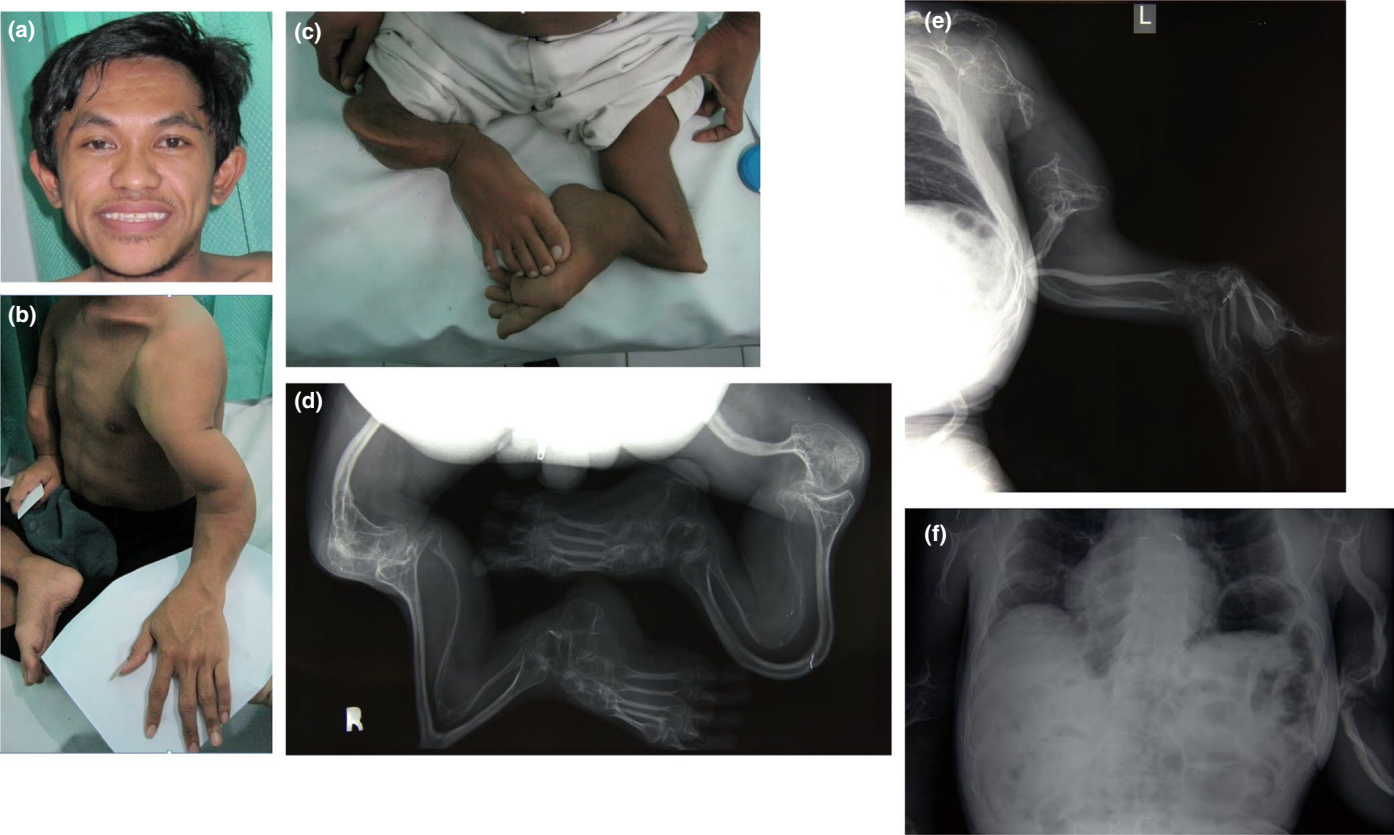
Clinical characteristics of the three affected brothers. (a) Facial features in patient II:5 showing normal sclerae and teeth. (b) Dysmorphic features in the left arm of patient II:5; the size of both hands is normal. (c) Severe bending of the femur, tibia, and fibula in patient II:5. (d) Radiological image in patient II:5 showing the skeletal malformations in the lower extremity. (e) Radiograph of left arm of patient II:5 revealing extensive resorption of the humeral shaft. (f) Radiograph of thoracic cavity in patient II:4 showing the appearance of wavy ribs.
